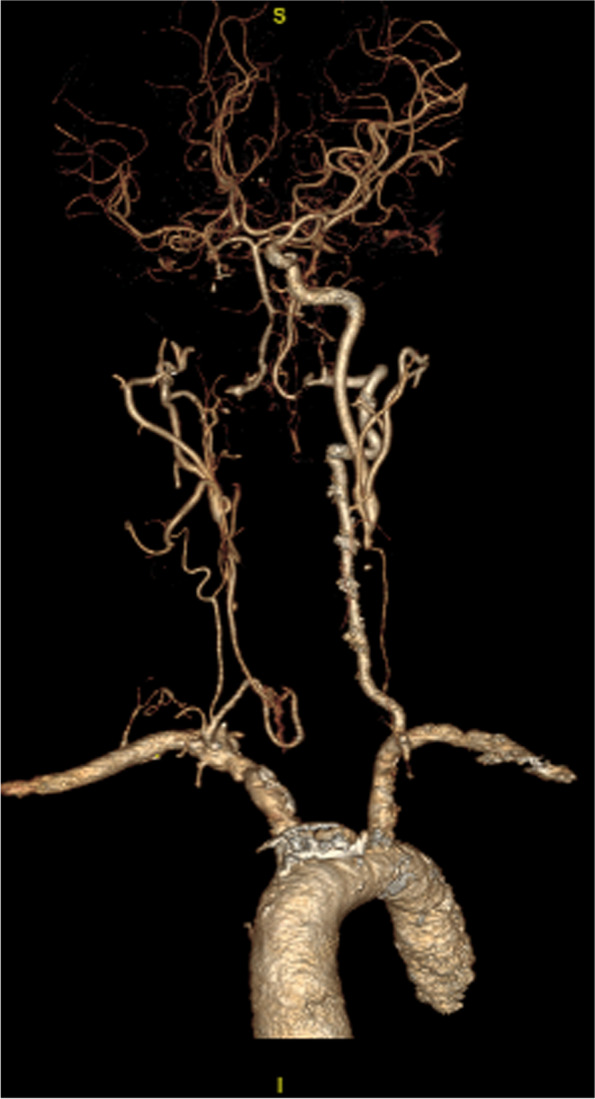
CTA. CTA showed nearly total occlusion of the bilateral CCA and nearly total occlusion of right ICA
